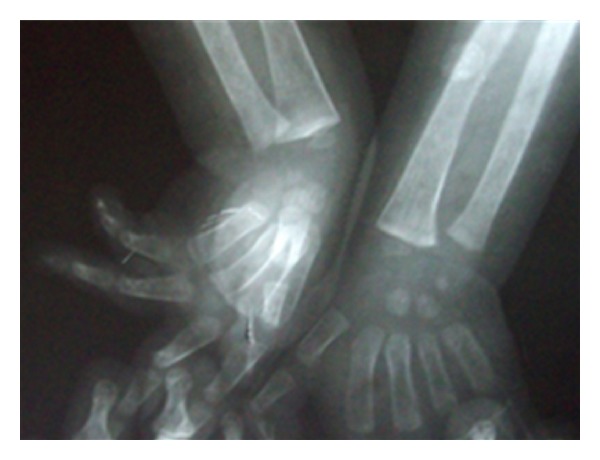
Showing wrist X-ray with features of healing rickets.
